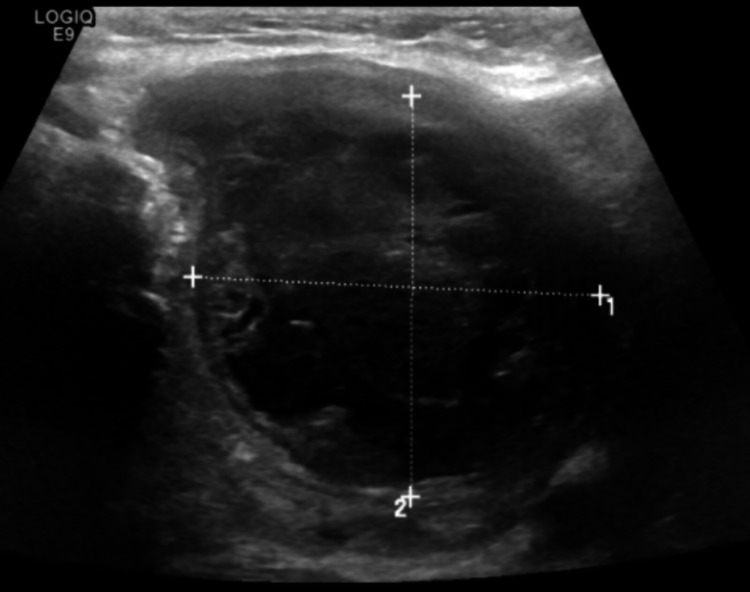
Ultrasound neck showed well-defined, large heterogeneous mass in left lobe.
